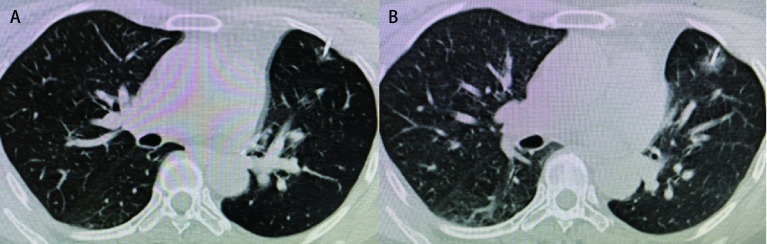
消融前后GGN的CT影像变化。A：消融前GGN；B：消融后肿瘤周围出现GGO，CT上靶区轮廓变得不清晰。 Comparison of CT images of GGNs before and after RFA. A: The image of GGN before RFA was performed; B: The tumor was covered by the GGO after RFA, and the contour of the target area becomes unclear on CT. GGO: ground-glass opacity.
